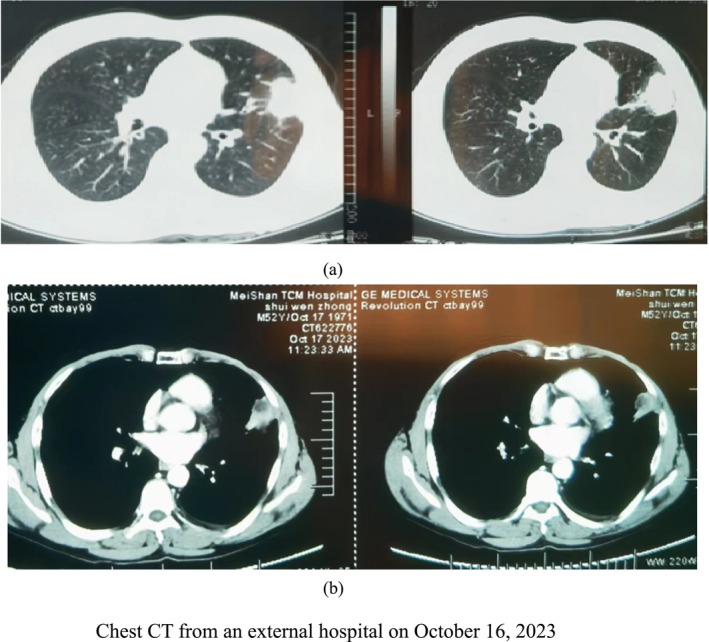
Chest CT from an external hospital on October 16, 2023.
